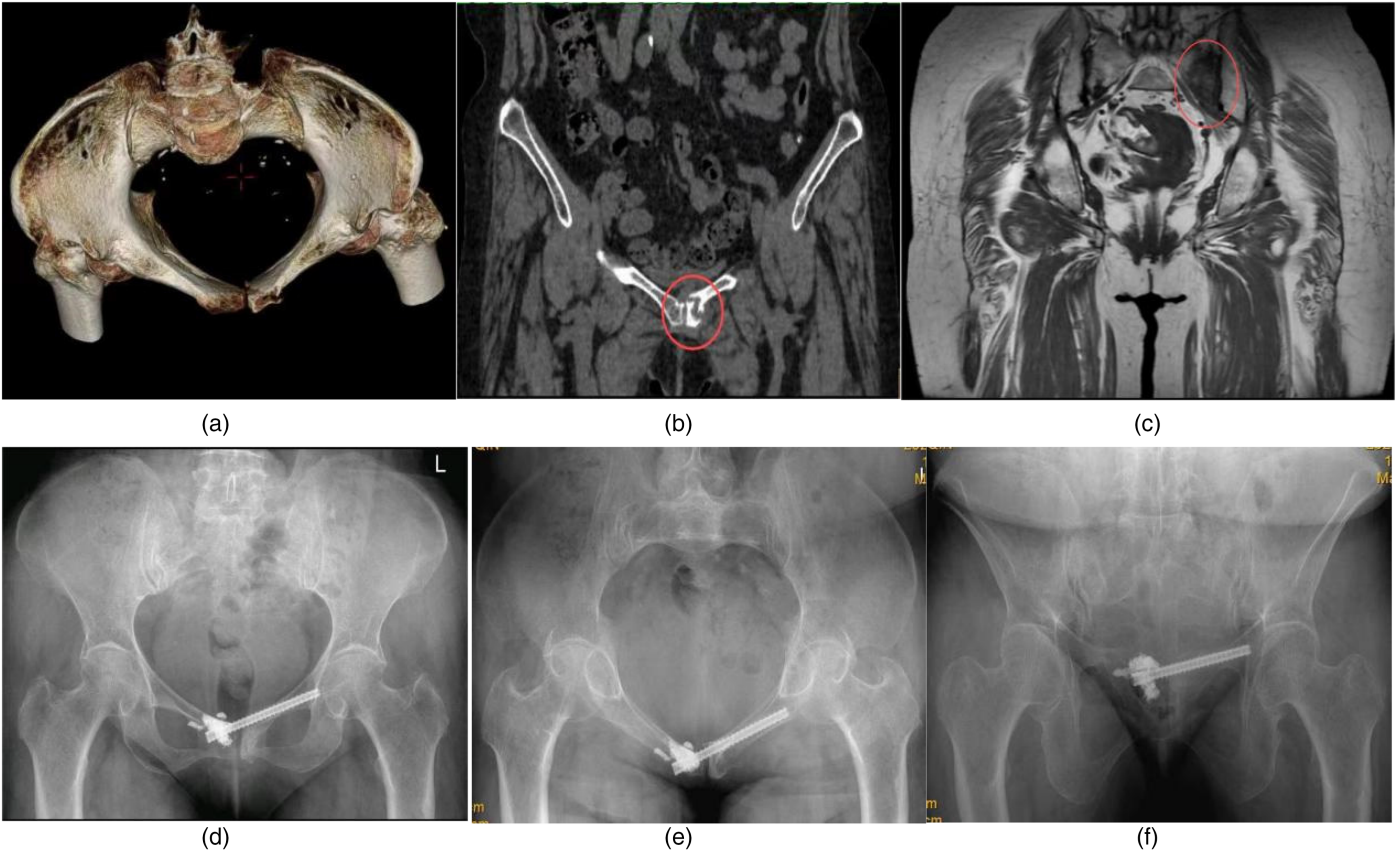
(a) Preoperative CT entrance view showed a fracture of the left pubic symphysis adjacent to the articular surface. (b) Preoperative CT scan showed sclerosis and nonunion of the symphysis pubis fracture. (c) Preoperative MR Showed that ipsilateral sacral alar bone marrow edema. (d) Postoperative anteroposterior pelvic images: cannulated screw fixation was performed across the pubic symphysis and bone cement reinforcement was performed at the end of the screw. (e) Screw position as shown in the postoperative pelvic entrance view. (f) The screw position as shown in the postoperative pelvic outlet view.
